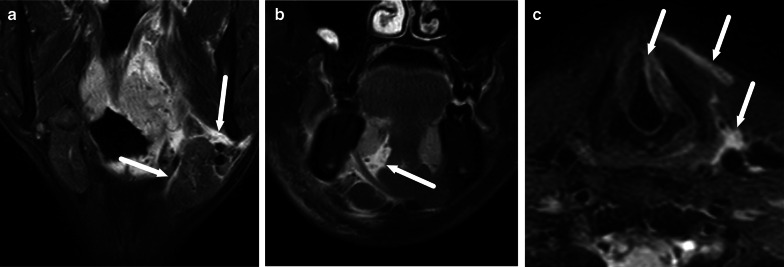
Examples of SMSE (a), SLSE (b), and VSE (c) on coronal (a, b) and axial (c) fat-suppressed T2-weighted images (arrows)
